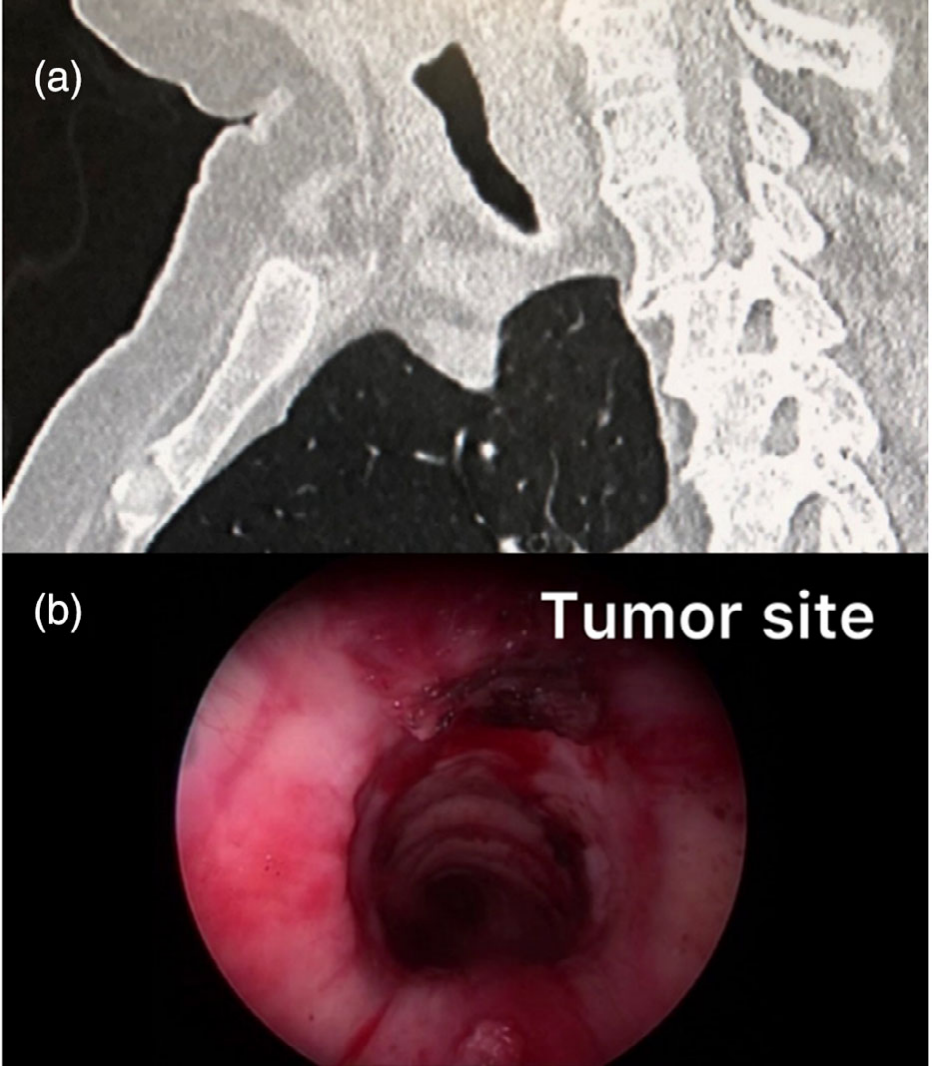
Endoscopy (a) and chest computed tomography (b) shows a recanalization of trachea after the procedure.
